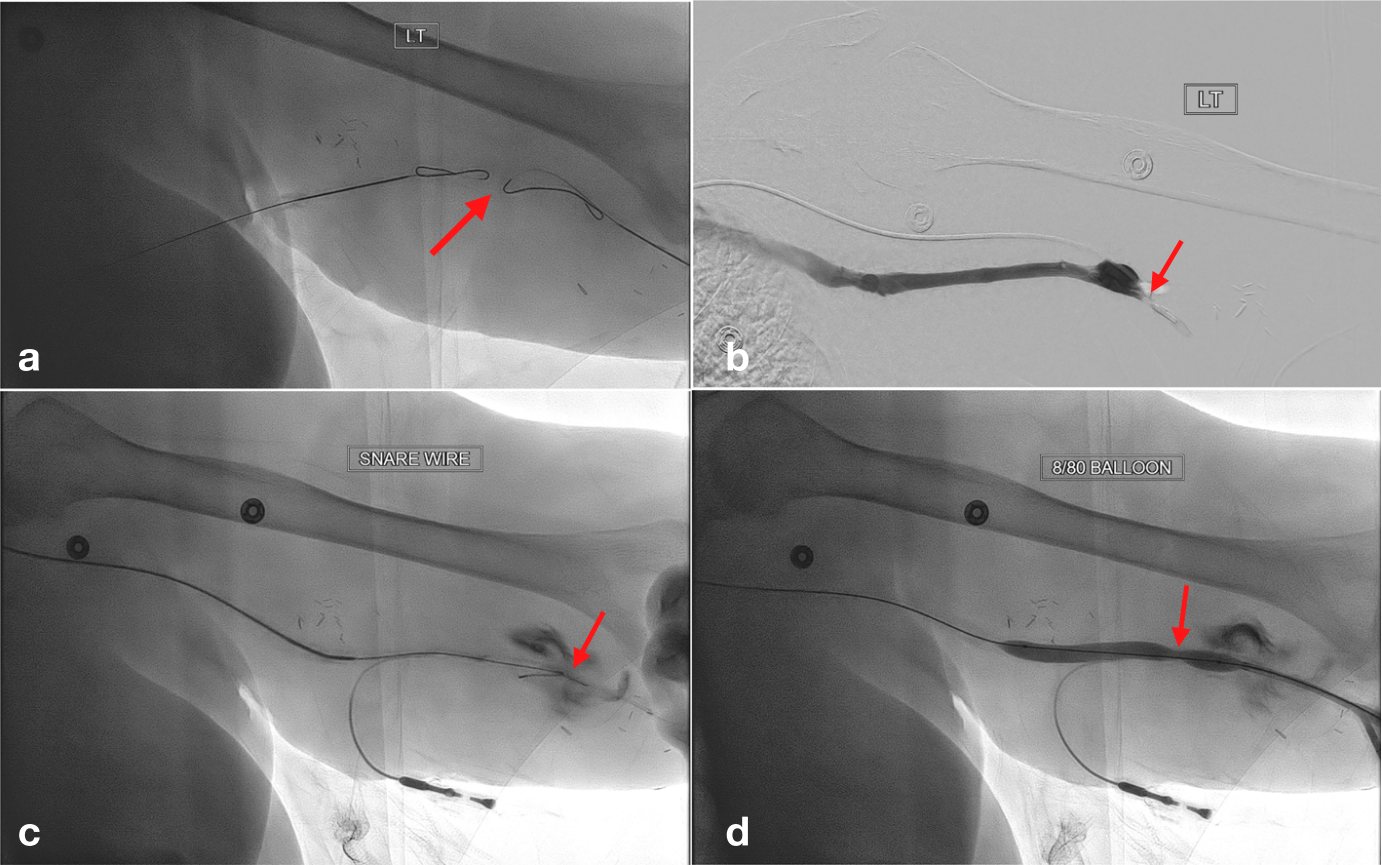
Failure to advance a 018″ guidewire viz. local antegrade and retrograde accesses due to focal obstruction (arrow) in the midportion of the graft correlating with area of delamination seen on ultrasound (a). Transfemoral access used to selectively catheterize the left brachial vein and contrast injection confirmed position within the graft vein anastomosis (arrow) (b). Four to eight millimeters microsnare via antegrade micropuncture sheath used to externalize the glidewire from the arm creating a through and through wire access (arrow) (c). Persistent flow defect is seen in the mid-portion of the graft (arrow) after Balloon angioplasty and platelet plug embolectomy of the graft-artery anastomosis demonstrating the area of delamination which needs to be grafted (d)
